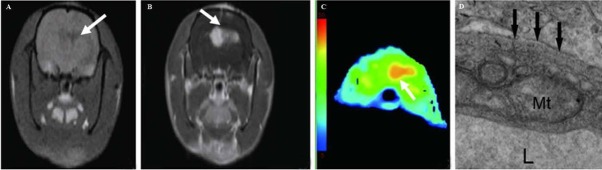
C6 glioma assessed with Gd-DTPA. A: T1-weighted image showed a hypointense tumor without distinct border (arrow). B: After administration of Gd-DTPA, A heterogeneous enhancement tumor with necrosis was noted (arrow). C: The mean of highest CBV areas(arrow) was 21.35 with administration of Gd-DTPA in the tumor. D: TEM image showed significant increase of the pinocytotic vesicles and opening tight junctions (arrow).
